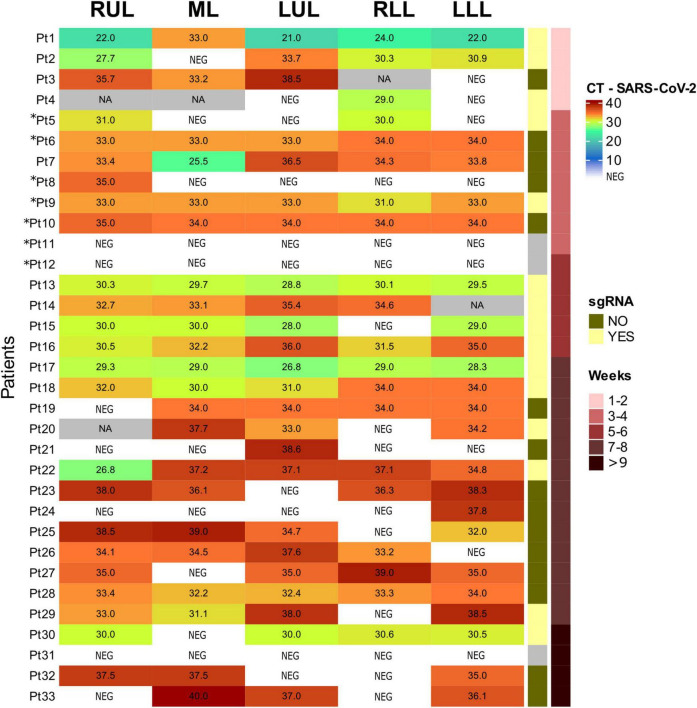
Sars-Corv-2 gRNA and sgRNA in lung samples. RUL, right upper lobe; ML, medium lobe; LUL, left upper lobe; RLL, right lower lobe; LLL, left lower lobe; CT, cycle threshold; NEG, negative; NA, non-available; sgRNA, subgenomic RNA. Patients not included in (17) are 5, 6, 8, 9, 10, 11, and 12 (with asterisks).
